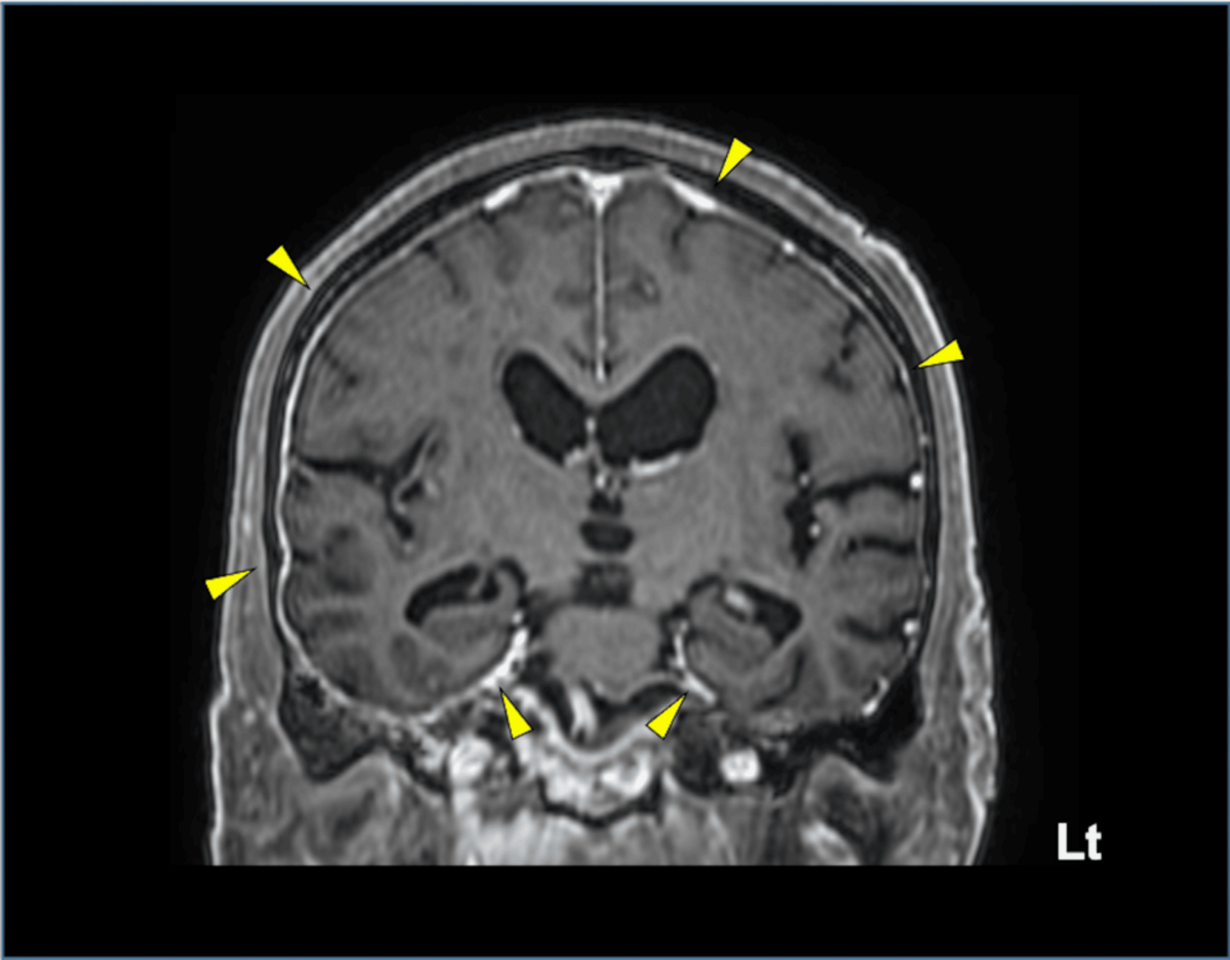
Gadolinium-enhanced T1-weighted brain MRI Diffuse dural thickening with enhancement evident bilaterally (yellow arrowheads).
